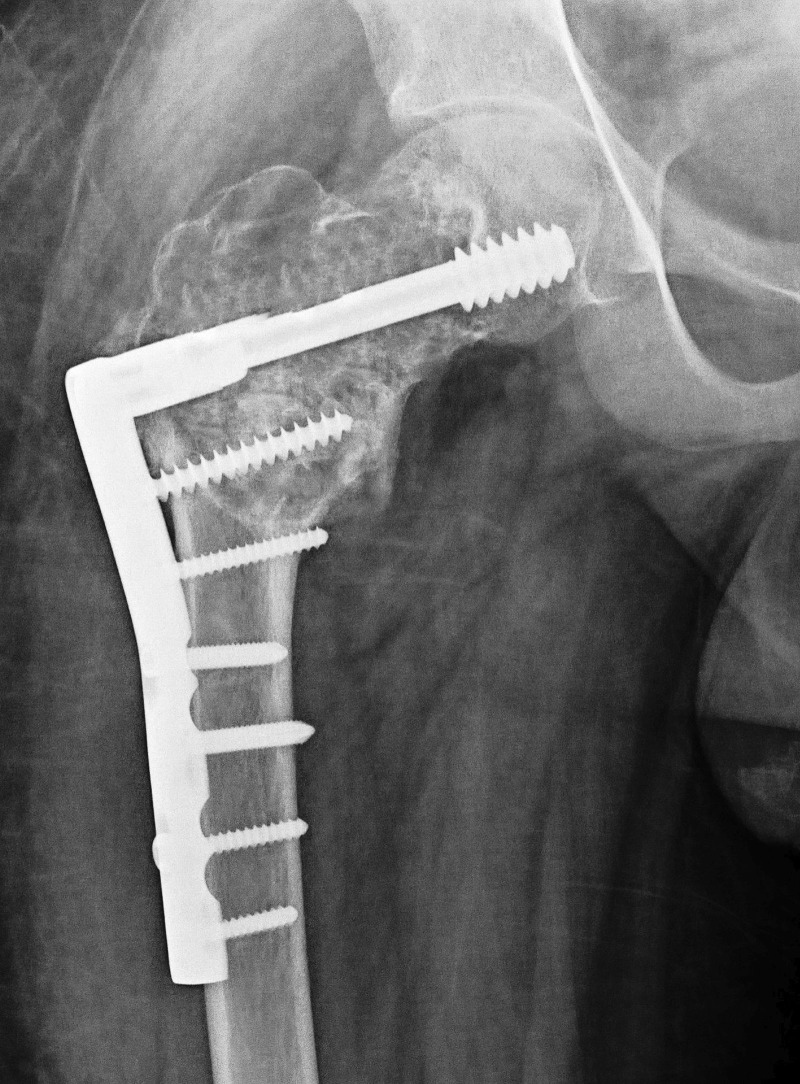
Anteroposterior X-rays of the hip with thigh at the one-year follow-up showing correction of the varus and improvement in the lytic lesion of the bone
